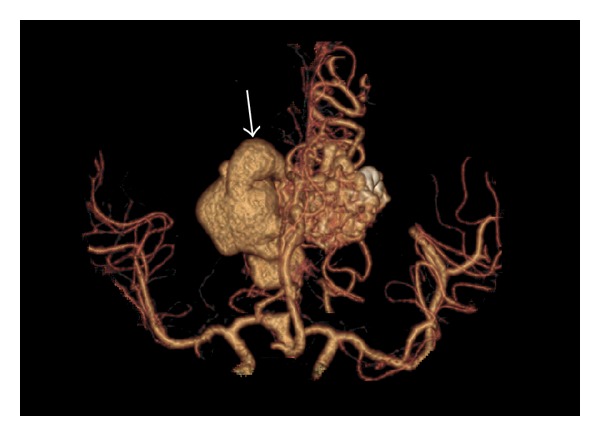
Axial contrast enhanced CTA (MIP) surface shaded image of the brain demonstrating a serpiginous intraventricular enhancing structure (arrow). The AVM is supplied by branches of the anterior cerebral artery and drains via superior sagittal sinus.
